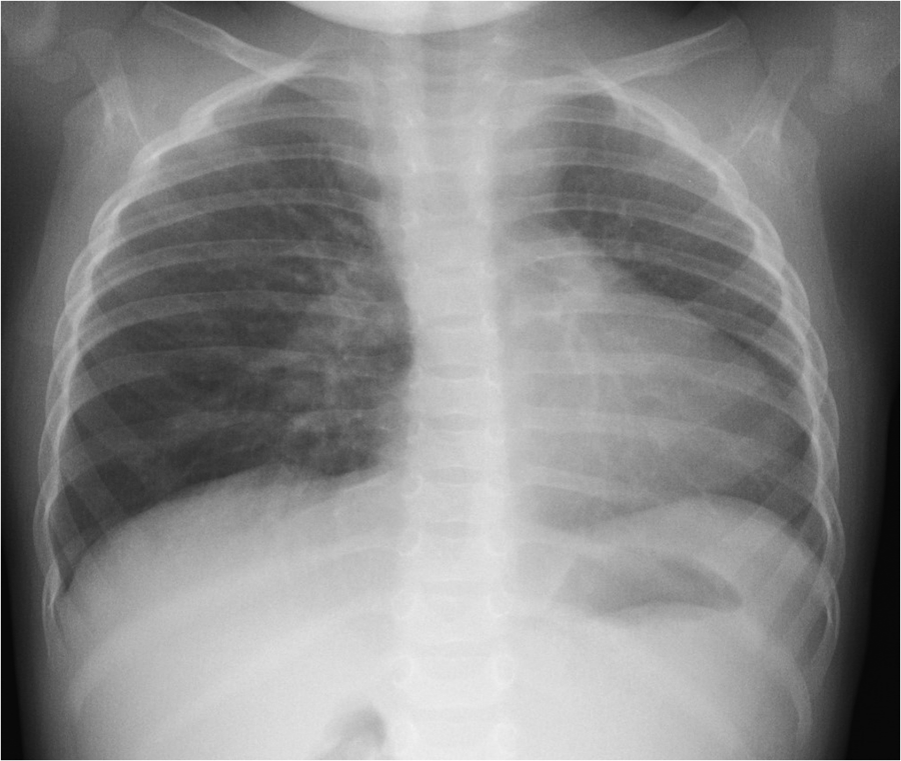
Chest radiograph image: decreased volume of the left lung, shifting of the mediastinum to the left, enlargement of the right lung hilum.
